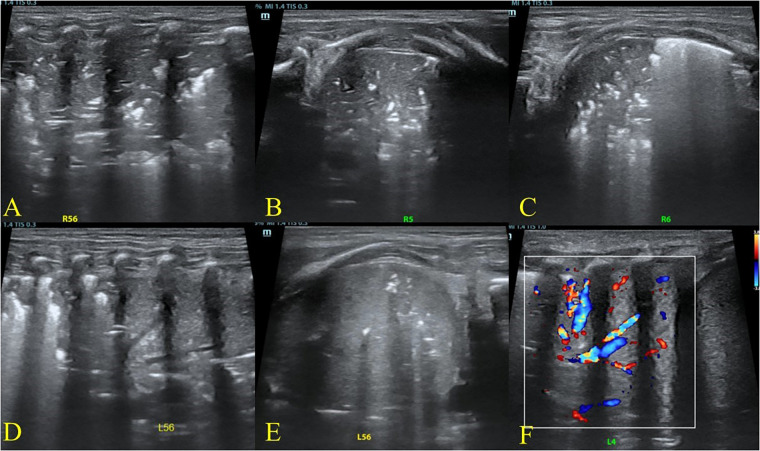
Baseline lung ultrasound findings at admission demonstrating bilateral posterior–lateral consolidation with intralesional vascularity. Initial ultrasound at admission demonstrated extensive consolidation in the lateral and posterior regions of both lungs. Air bronchograms were more prominent on the right than on the left. Color Doppler revealed vascular signals within the consolidated areas, consistent with pneumonia. (A–C) Longitudinal and transverse views of the right lung, zones 5–6; (D–E) longitudinal and transverse views of the left lung, zones 5–6; (F) color Doppler showing vascularity within the consolidated region.
